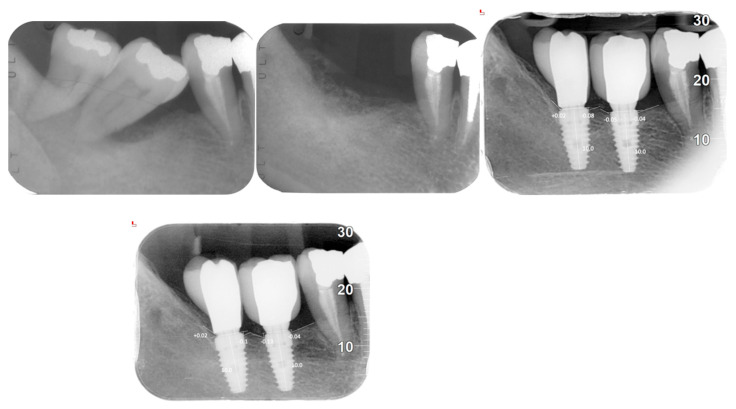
X-ray follow-up during the time. Extractions from 2019, 2021, and 2023.
